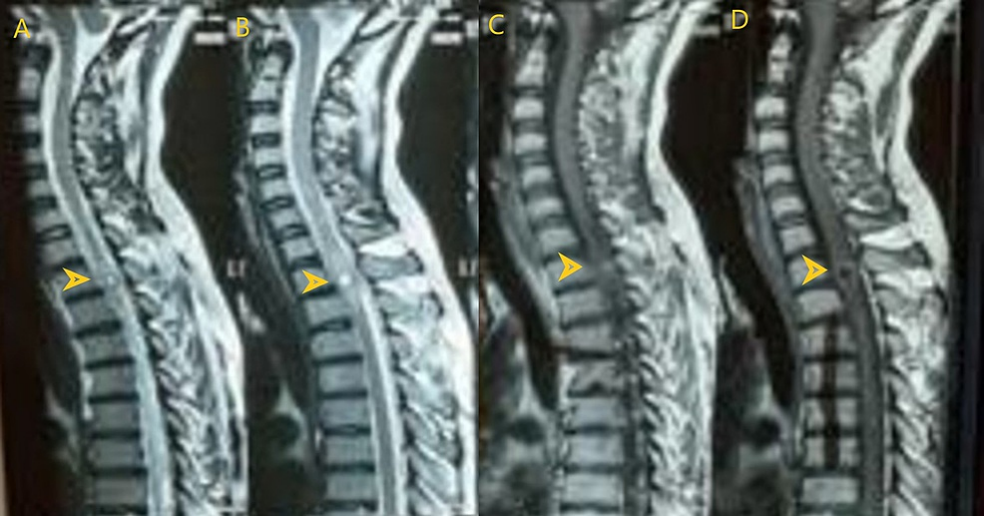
Cervico-dorsal spinal MRI. (A) Short TI-inversion recovery image. (B) T2-weighted image. (C) Post-contrast T1-weighted image. (D) Plain T1-weighted image.
